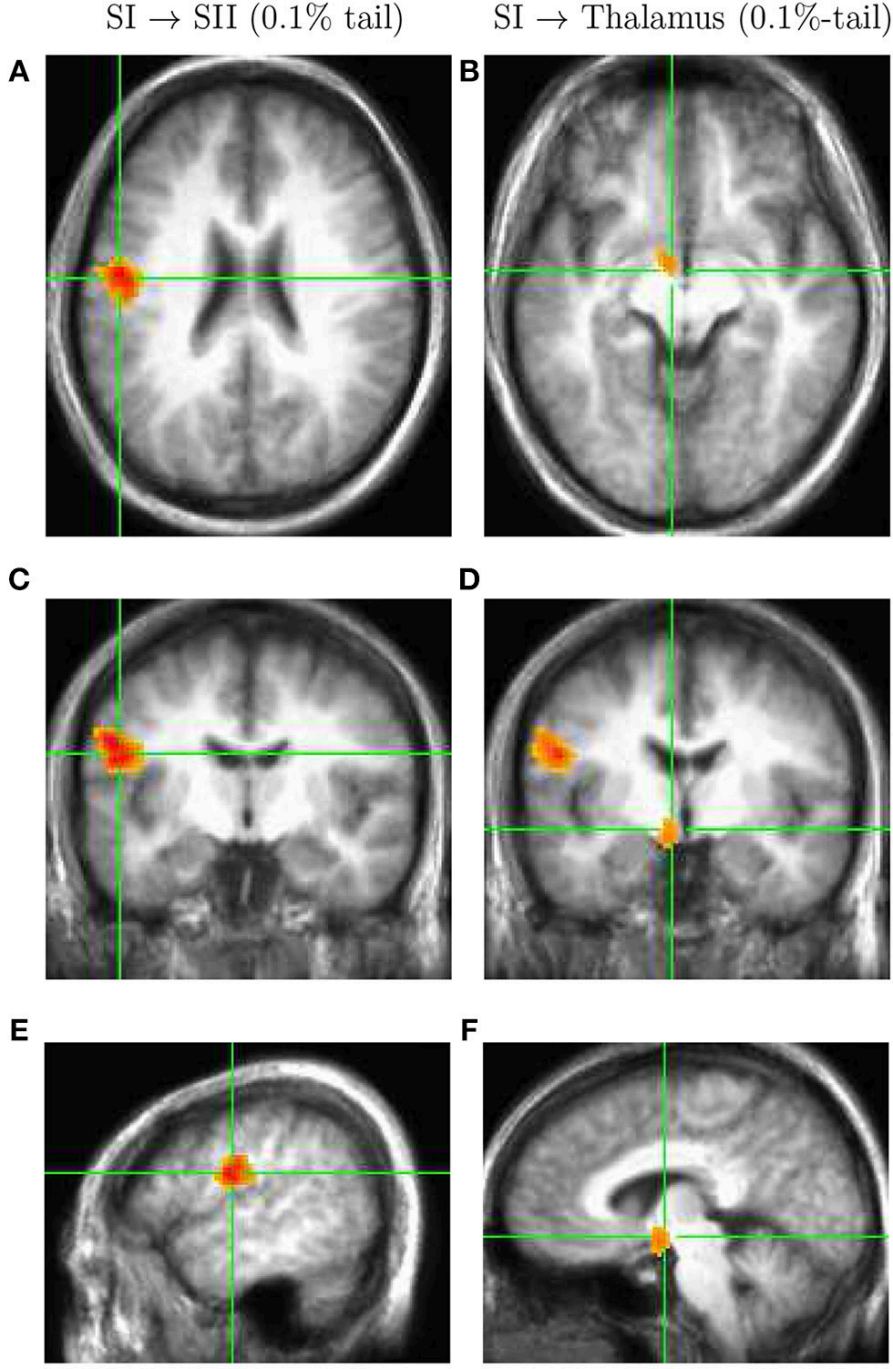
Volumetric map of net information transfer with respect to the primary somatosensory area SI. The map is thresholded at the 0.999-quantile (0.1% tail in Figure 2), and is superimposed on subject-averaged anatomical MRI. Two clusters of virtual channels are identified: secondary somatosensory area SII (left column, A,C,E), and a thalamic source (right column, B,D,F). The directionality of dominant information transfer is from SI to SII and thalamus.
